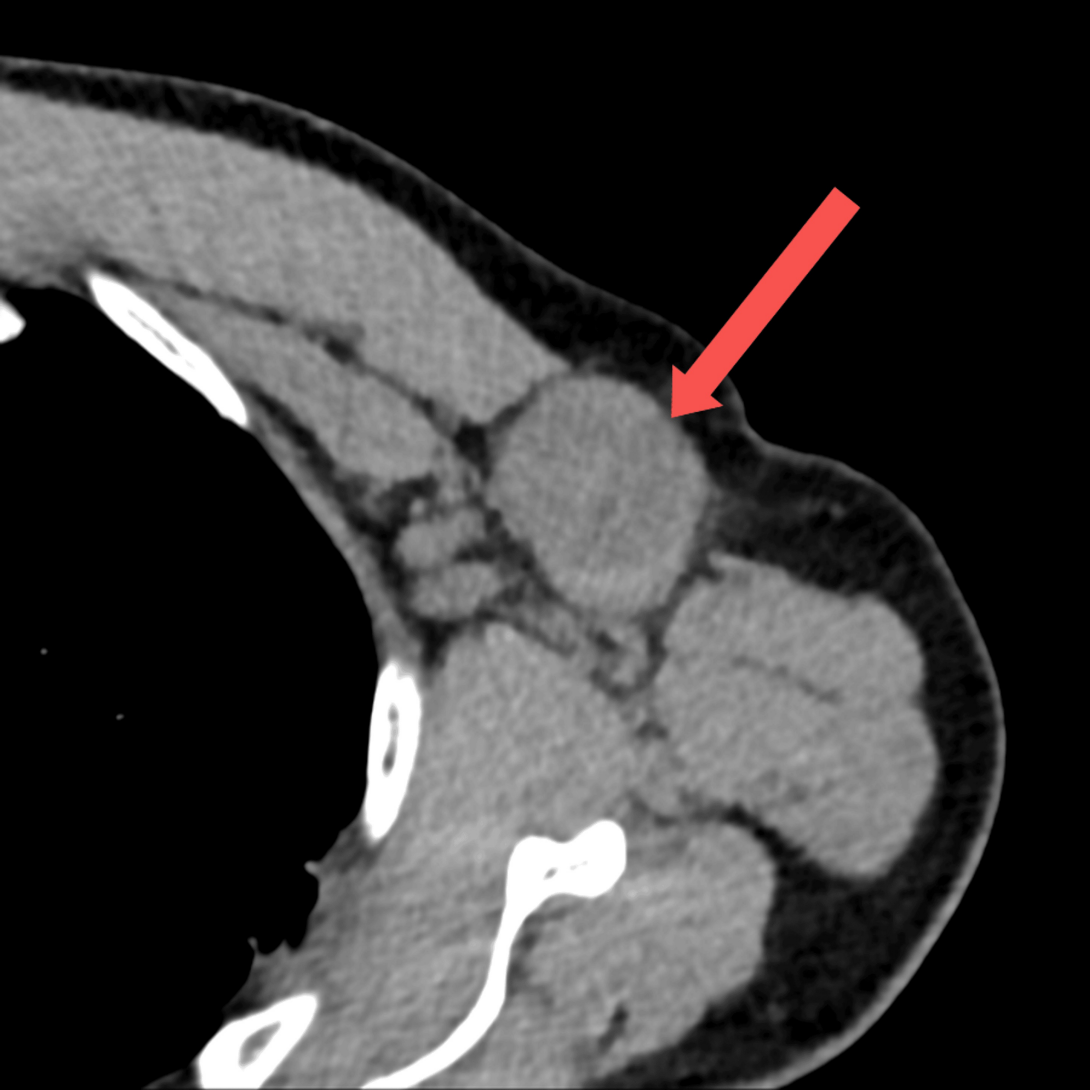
Preoperative computed tomography image of the left axillary mass.
